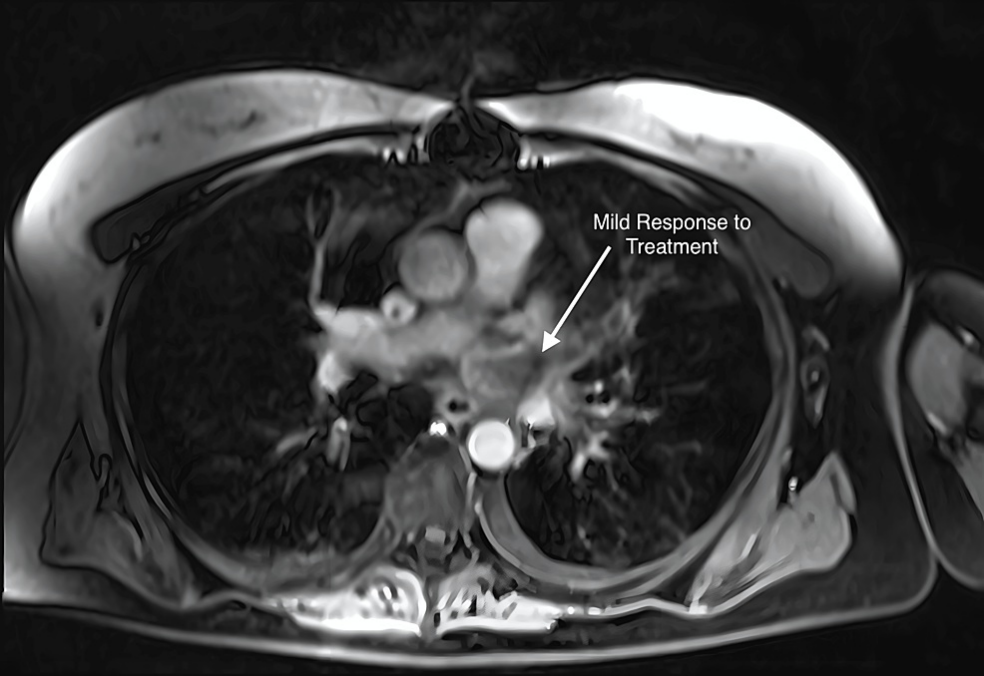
Axial MRI performed 3/20/2020 demonstrating partial response of the cardiac sarcoma.
